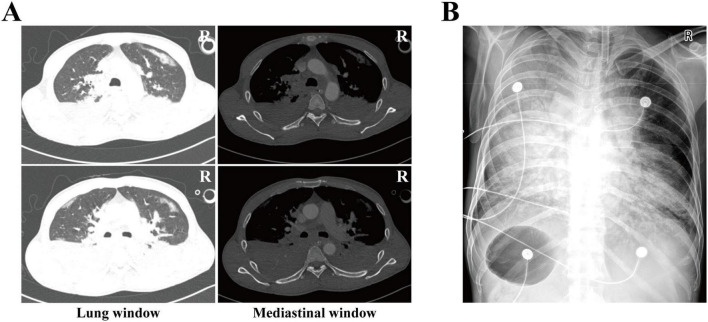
Imageological examination. (A) Chest CT on admission. (B) Bedside DR two days after admission. CT Computed Tomography, DR digital radiography.
